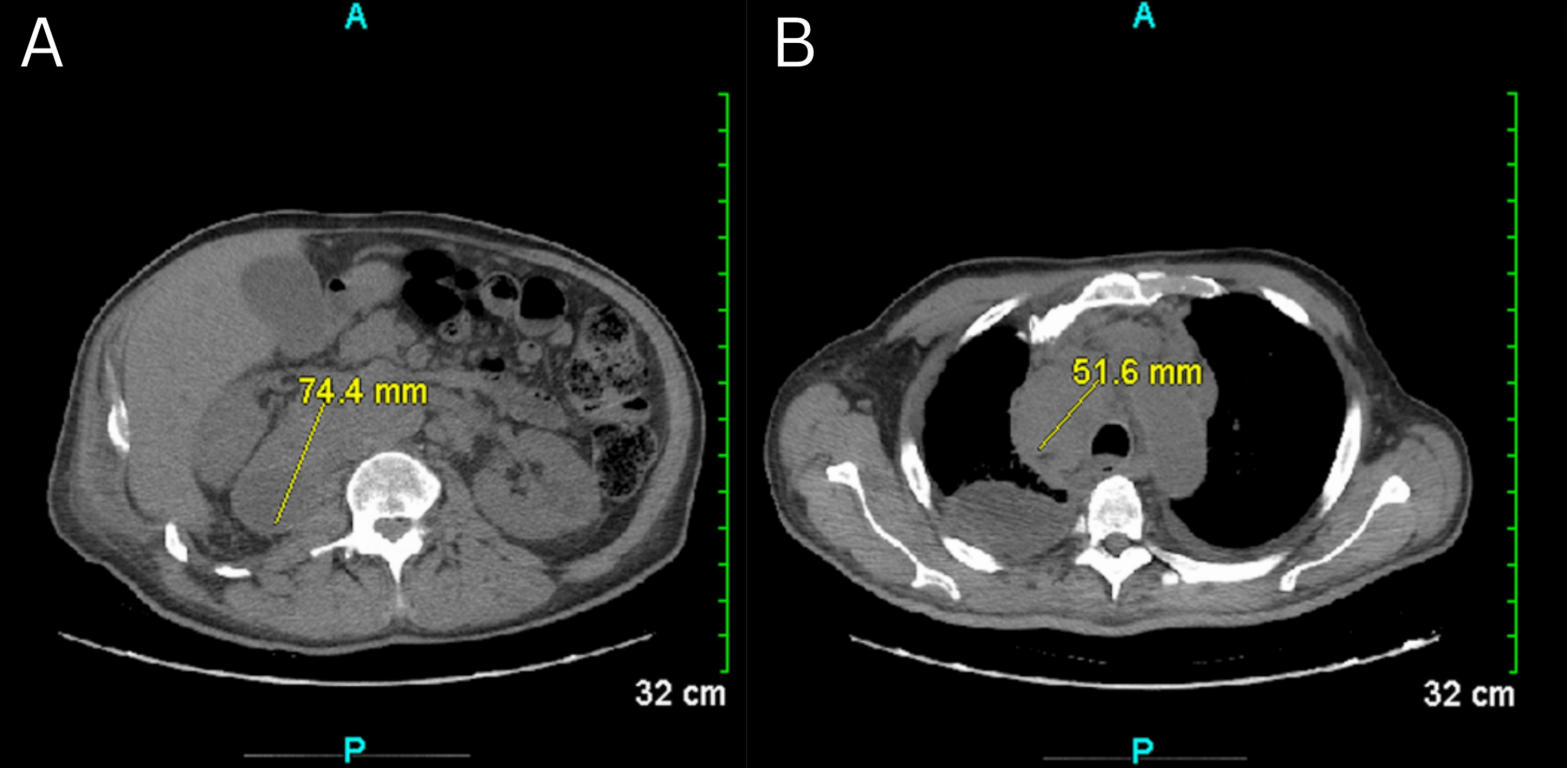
The CT scan shows a mass within the right adrenal gland that extends inferiorly and medially, displacing the right kidney, with a measurement of at least 7 cm (A). There is abnormal soft tissue in the right paratracheal region, consistent with lymphadenopathy, measuring at least 5 cm (B). Additionally, extensive atelectasis in the right lung obscures the right hilum, and a small left pleural effusion is also observed. CT: computed tomography.
